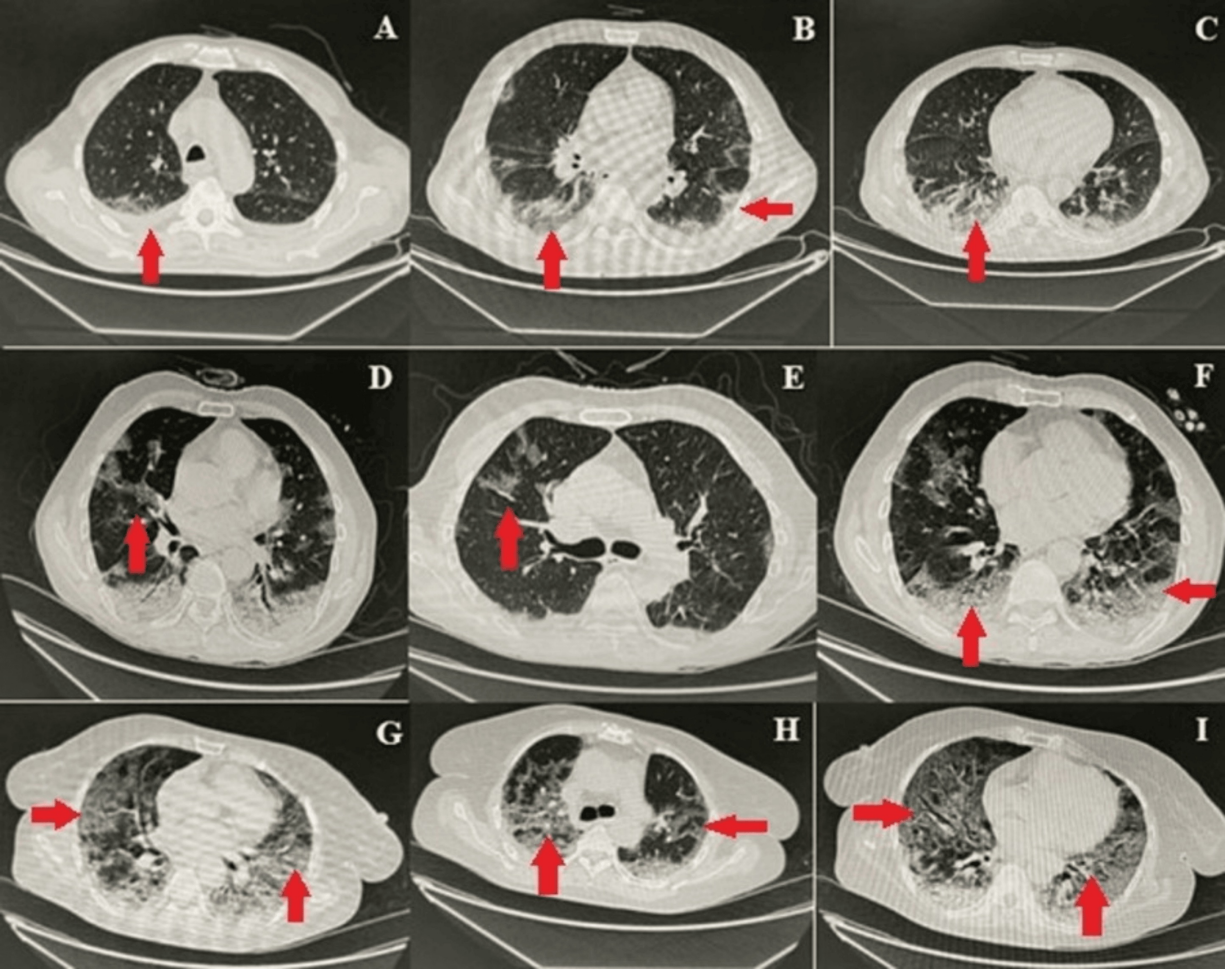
Representative chest CT images showing COVID-19 pneumonia Images A-I show different patterns of opacifications and infiltrates (arrows) ranging from ground glass opacities (e.g., image I) to lobar consolidations (e.g., image D) associated with COVID-19 pneumonia Panels A, B, and C in the figure show COVID-19 pneumonia with pulmonary involvement of 30-40% CO-RADS-6 with chest CTSS-mild. There are bilateral minimal pleural effusion, bilateral peripheral, and basilar ground glass opacities, subpleural reticulations, and consolidations in lower lobes; multiple enlarged mediastinal lymph nodes are seen in the left para-aortic and subcarinal regions. Included sections of the stomach showed circumferential thickening of antrum and pylorus with mural thickness up to 3 cm in the anterior wall. There was about 5-7 ´5.2 cm well-defined soft tissue density lesion inseparable from the tail of the pancreas. Panels D, E, and F show atypical pneumonia COVID-19, CO-RADS-6 with chest CTSS (15/25) (moderate Pulmonary involvement). There are patchy areas of ground glass haze with peripheral predominance in bilateral upper lobes, middle lobe, and lingula. Apical and basal segments of both lower lobes show consolidations with air bronchogram. Panels G, H, and I show atypical pneumonia COVID-19, CO-RADS-5 with radiological chest CTSS (19/25) (severe pulmonary involvement). There are multifocal patches of ground glass haze, and consolidations are seen in both lung fields with peripheral and basal predominance CO-RADS: COVID-19 Reporting and Data System; COVID-19: coronavirus disease 2019; CT: computed tomography; CTSS: computed tomography severity score
